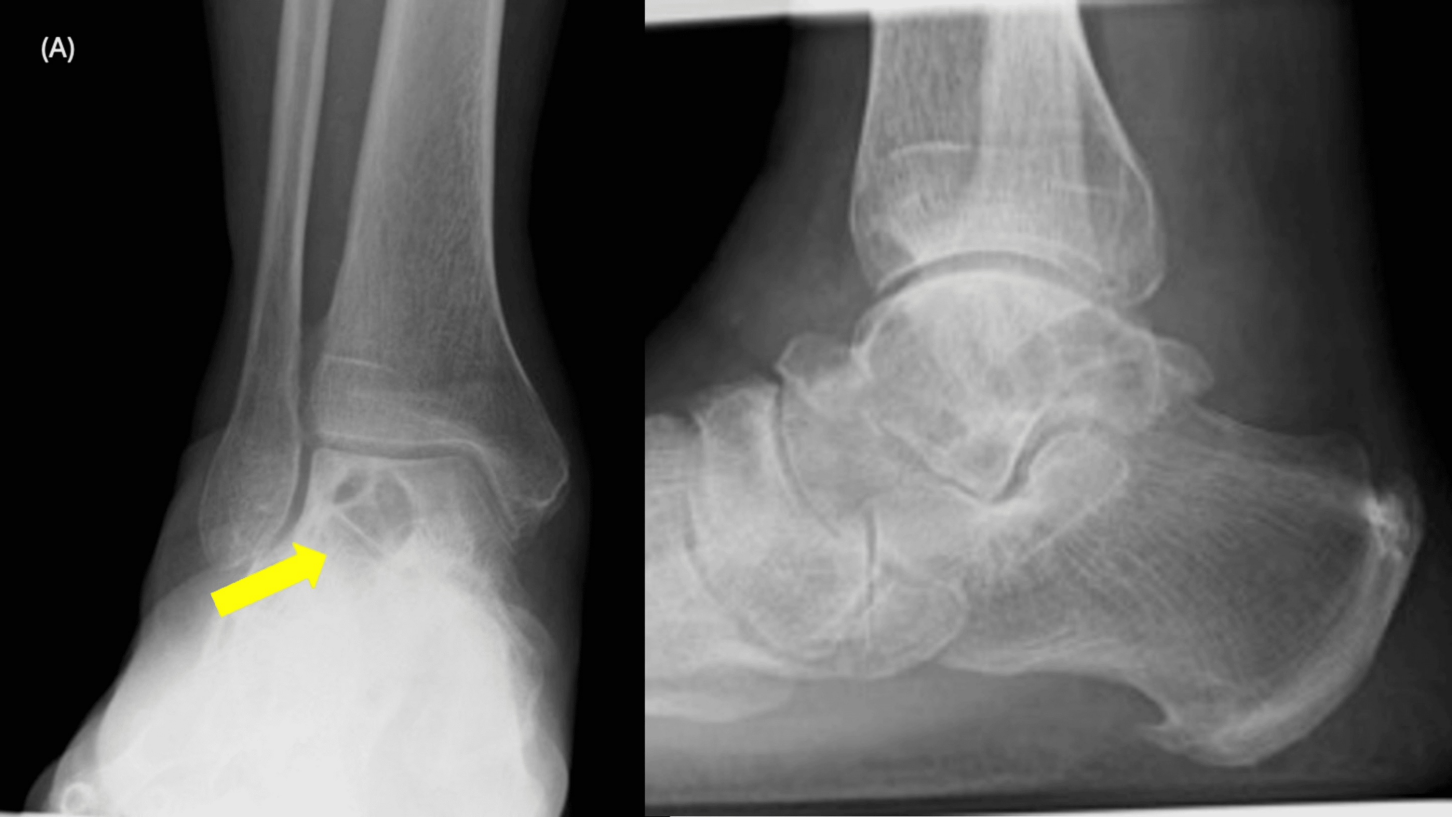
Preoperative radiographs indicating osteolytic lesion in the talus (yellow arrow)
